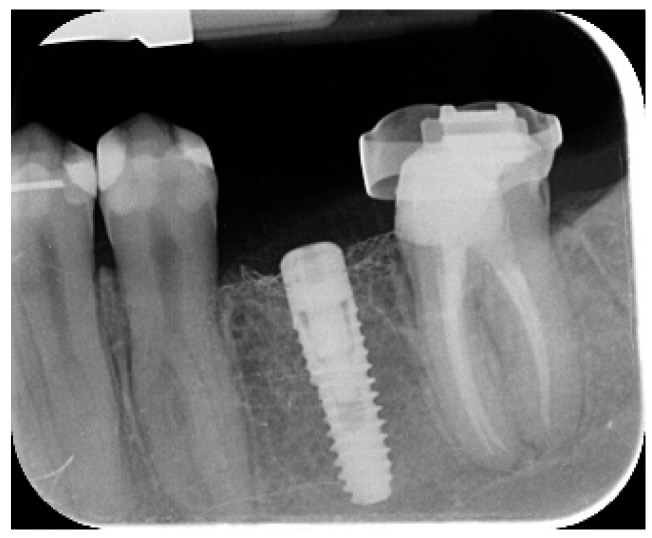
Radiographic view at implant placement.
